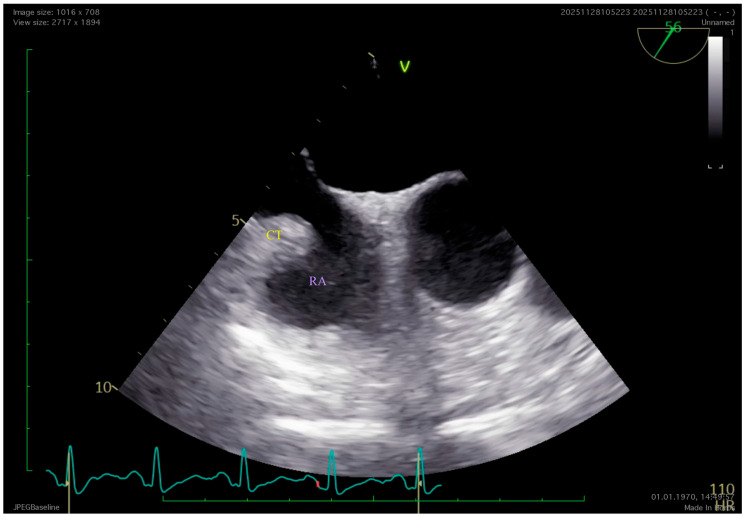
TEE showing a hypertrophied crista terminalis presenting as a right atrial mass. CT: crista terminalis, RA: right atrium.
